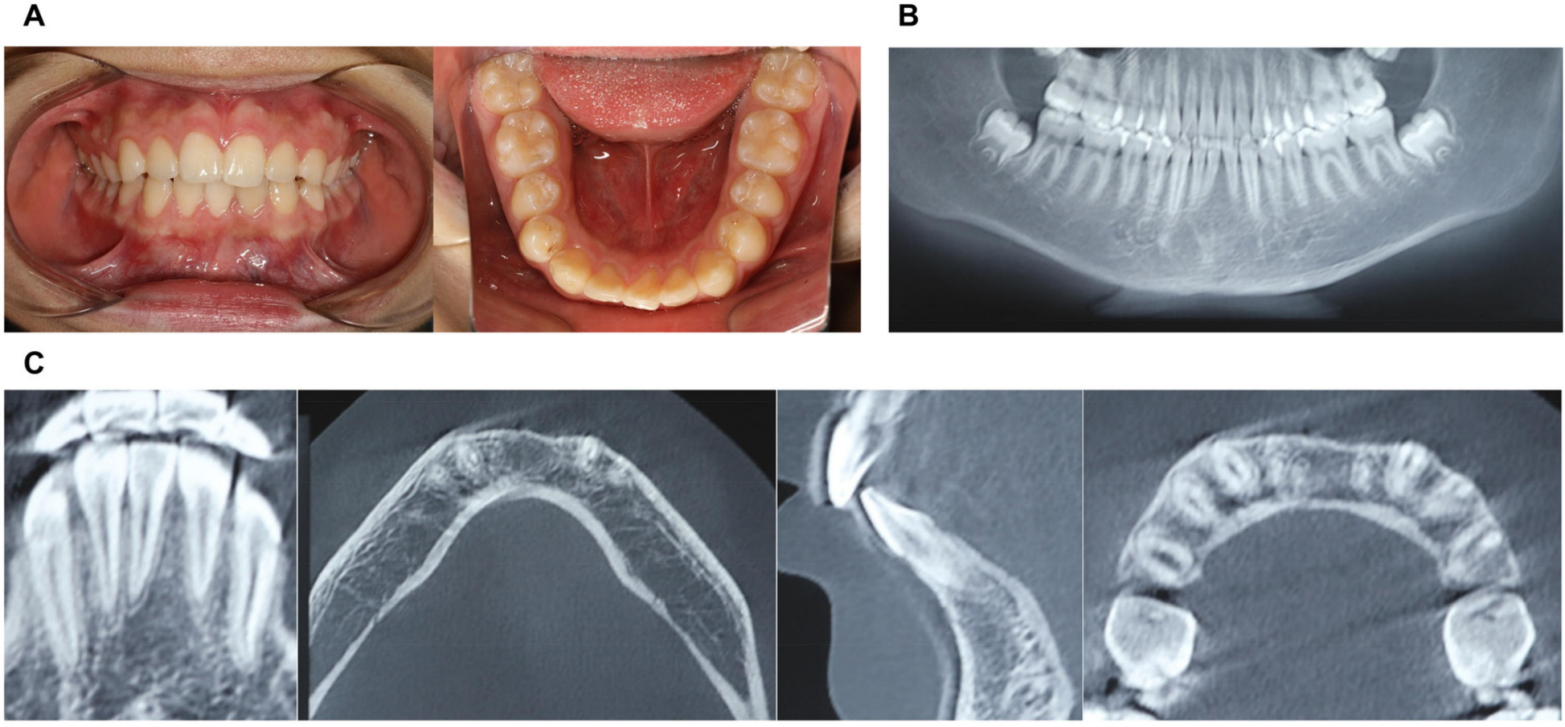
Physical and CBCT examination one year after surgery. (A) Photos of intraoral view; (B) CBCT examination (overall view); (C) CBCT examination.
